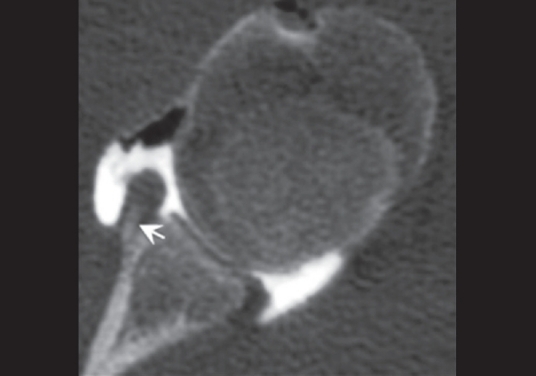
Axial CT-Arthrography image of a 30-year-old man with arthroscopy proved ALPSA lesion and demonstrates the periosteal reaction (*arrow*) due to periosteum stripping
